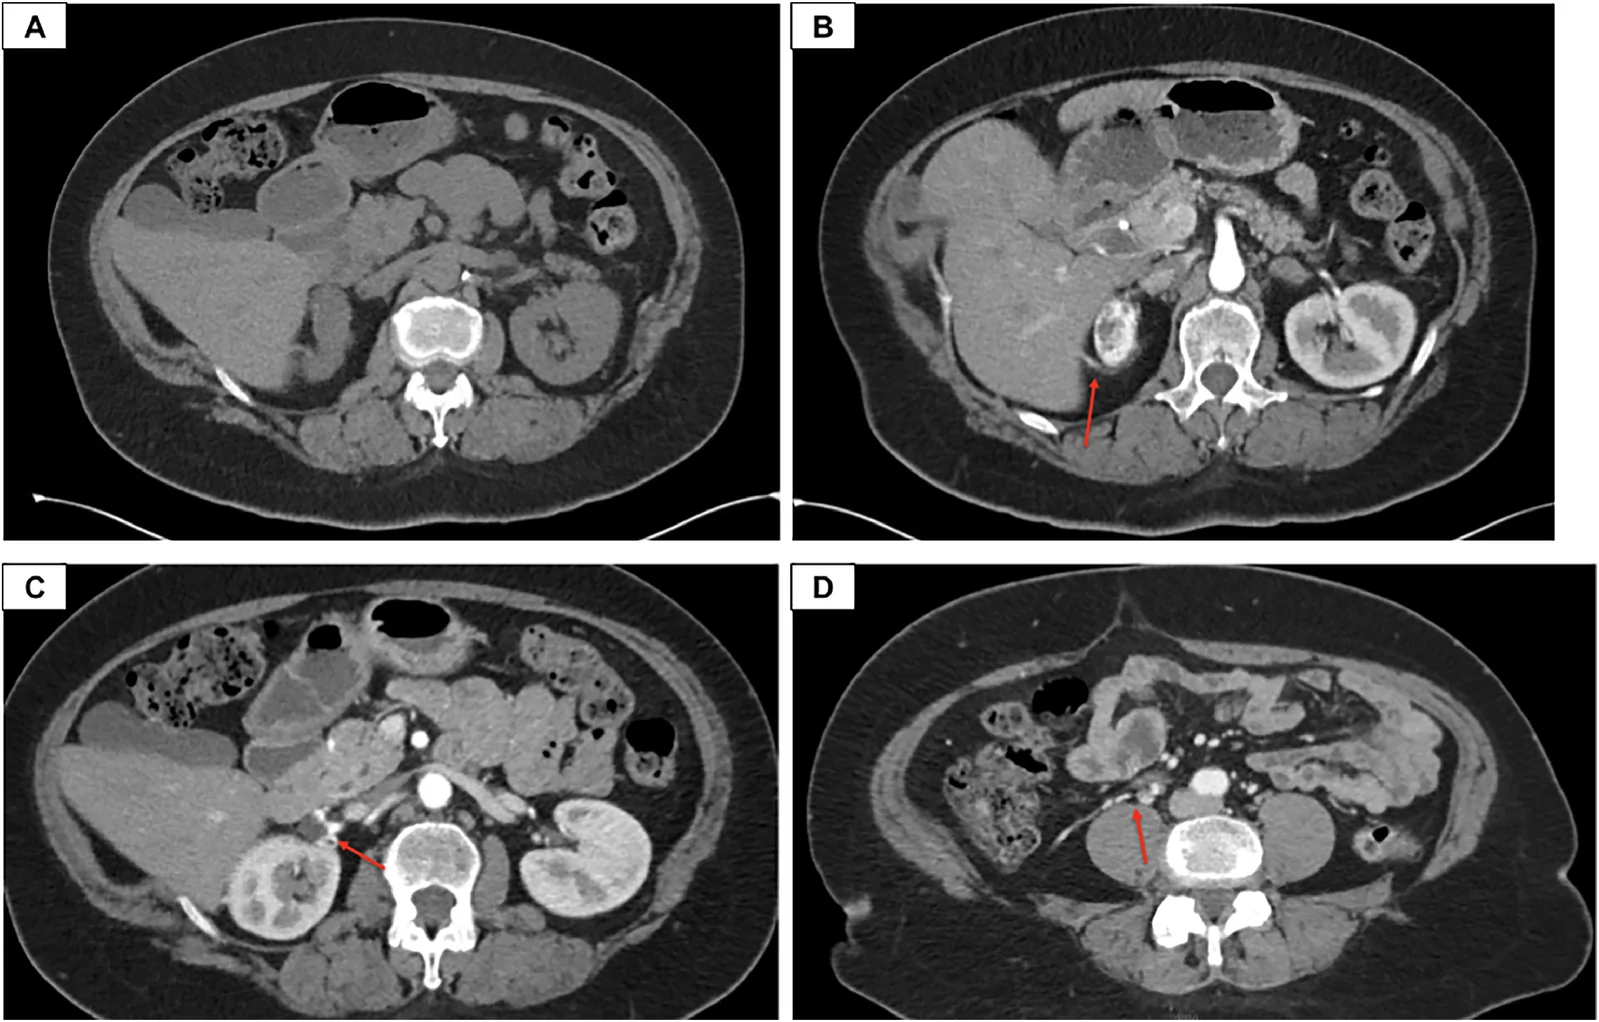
Multiphase CT of the right perirenal mass. (A) Noncontrast CT demonstrates the lesion without internal calcification. (B) Arterial-phase CT demonstrates early enhancement near the right renal hilum and rapid enhancement of the draining vein (arrow). (C) Arterial-phase CT demonstrates arterial supply from the right main renal artery (arrow). (D) Venous-phase CT demonstrates drainage through a renal capsular vein into the right ovarian vein (arrow).
